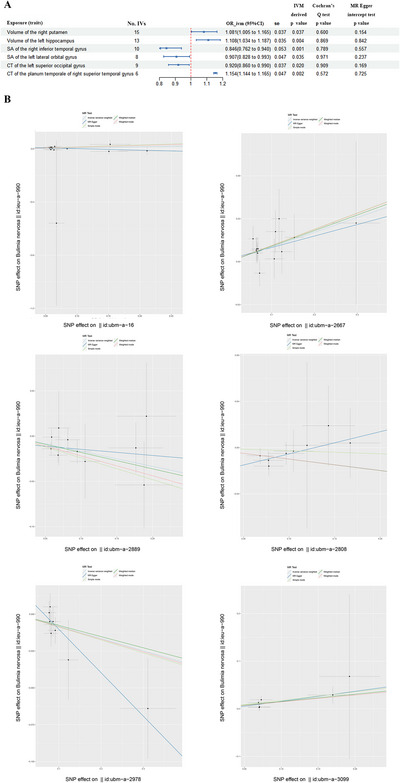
Significant findings from the reverse Mendelian randomization (MR) analysis of bulimia nervosa on brain structural traits. And *p* < 0.05 was regarded as suggestive potential causality. (A) Six brain structural traits associated with the risk of bulimia nervosa in reverse MR analysis. (B) Five analysis methods in the reverse MR analysis of the above six structural traits. CI, confidence interval; CT, cortical thickness; IVW, inverse‐variance weighted; No. IVs, number of instrumental variables; OR, odds ratio; SA, surface area; se, standard error; SNP, single‐nucleotide polymorphisms.
